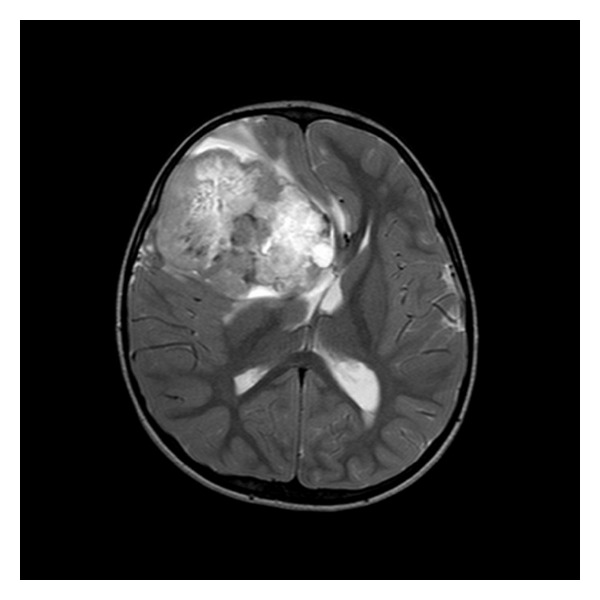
MRI brain: large right frontal lobe mass.
